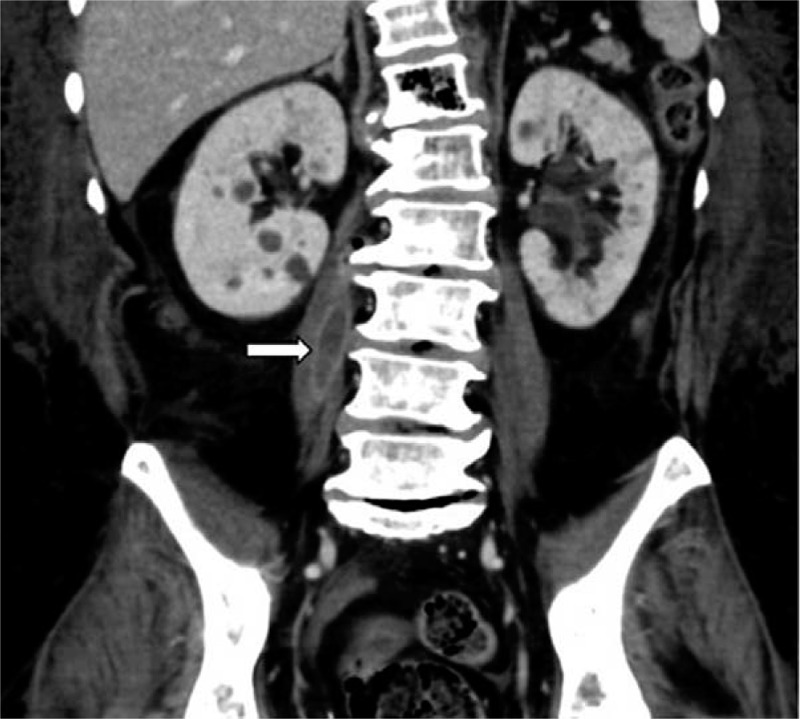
Follow-up computed tomography scan in coronal plane showing newly developed right psoas abscess at L1-L4 level. (arrow).
